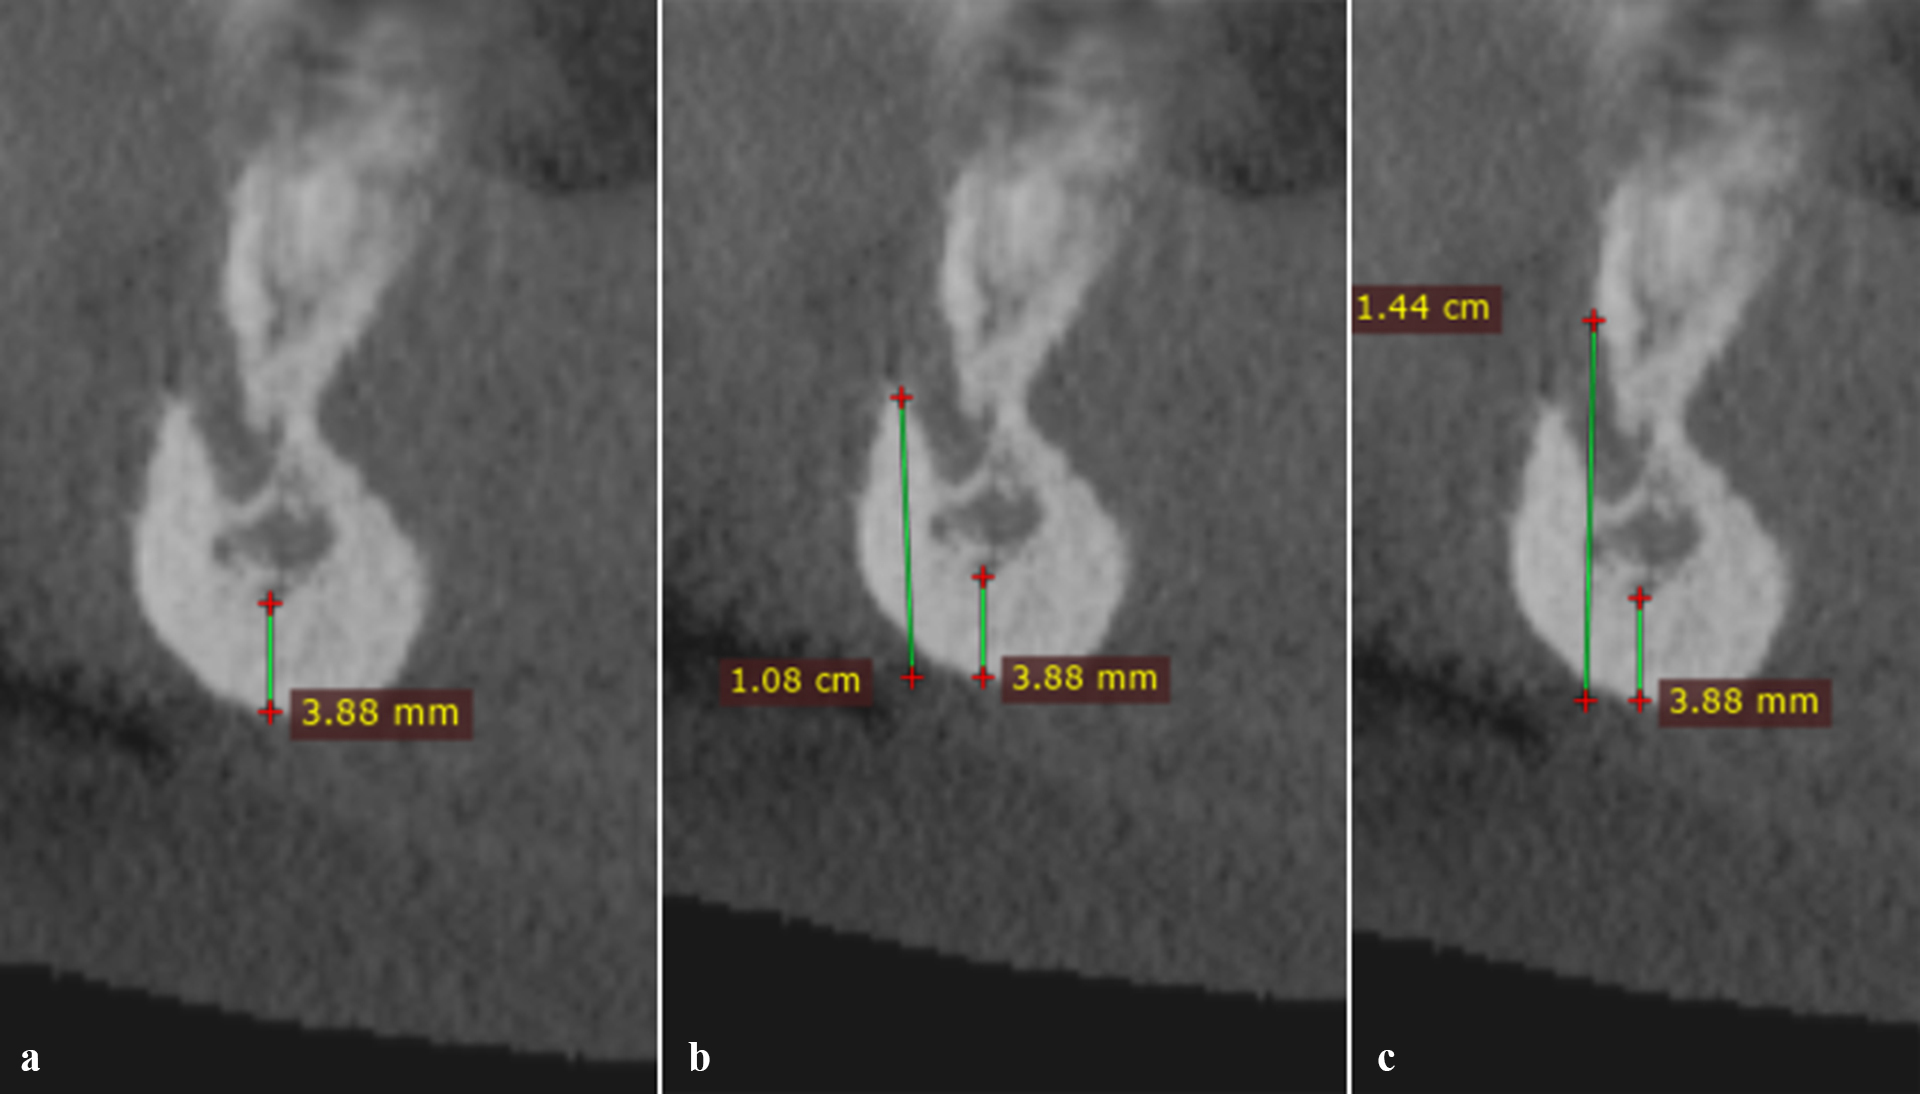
Analyzed indexes in cone beam computed tomographic images: (a) computed tomography mental index, (b) computed tomography index (inferior), and (c) computed tomography index (superior)
